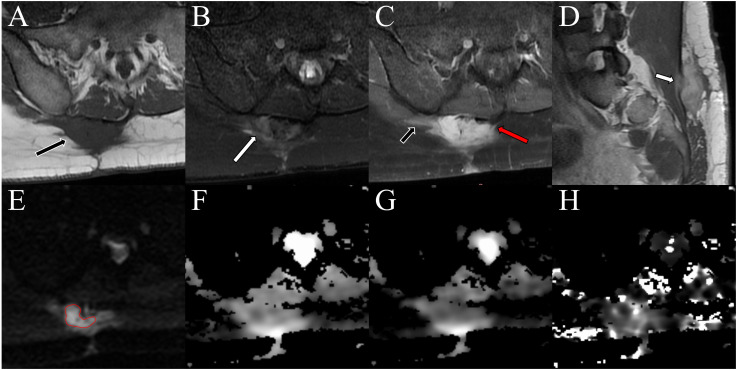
A 32-year-old patient with desmoid-type fibromatosis of the back. (A) Axial T1-weighted image showed an irregular hypointense mass (long black arrow). (B) Axial T2-weighted images showed a heterogeneous mass with a band sign (long white arrow), extending towards the skin area. (C, D) Contrast-enhanced T1-weighted image showed heterogeneous enhancement of the lesion, invasion into the surrounding fat space and erector spinae (short white arrow), and presentation of fascial tail sign (long red arrow) and peritumoral enhancement (short black arrow). (E) The solid portion of the lesion showed marked hyperintensity on the IVIM (b = 800 s/mm^2^) image; (F) the standard-ADC_mean_ and standard-ADC_min_ values were 1.29 × 10–^3^ mm^2^/s and 1.06 × 10–^3^ mm^2^/s; (G) the D_mean_ and D_min_ values were 1.18 × 10–^3^ mm^2^/s and 0.83 × 10–^3^ mm^2^/s; and (H) the f_mean_ and f_min_ values were 17.70 and 7.47.
